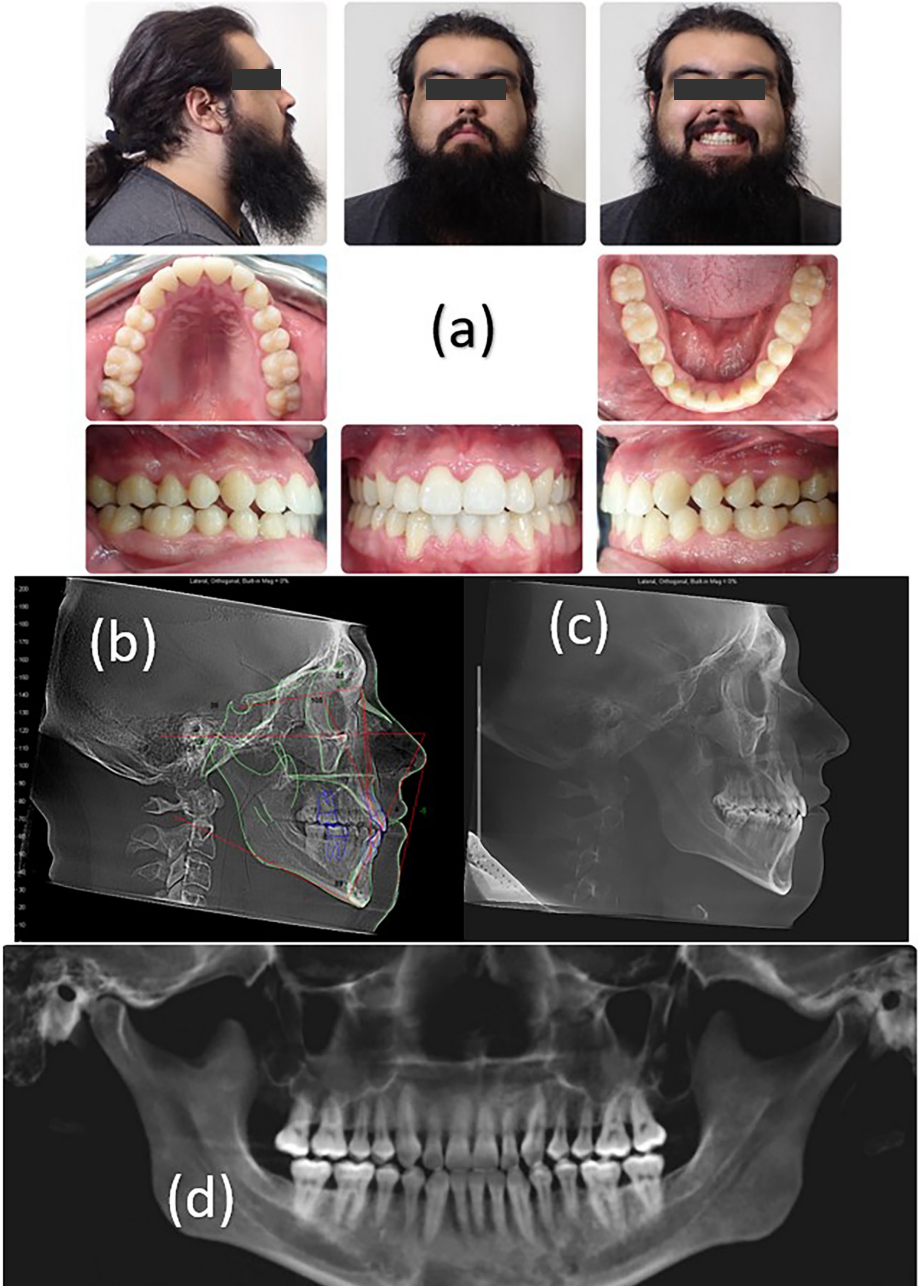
(a) Post treatment facial and intraoral photographs, (b) Post treatment cephalogram, (c) tracing and (d) panoramic radiograph.
